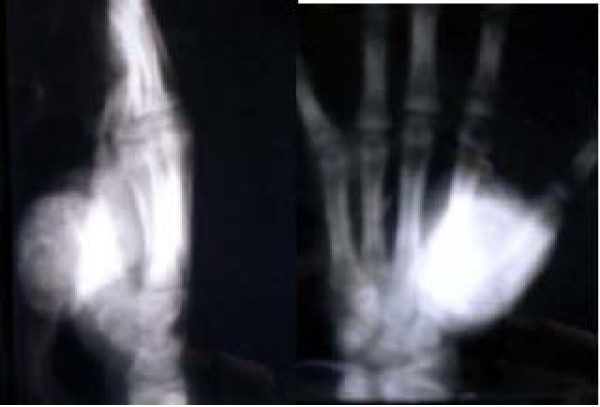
AP & Lateral radiograph of hand of our patient
